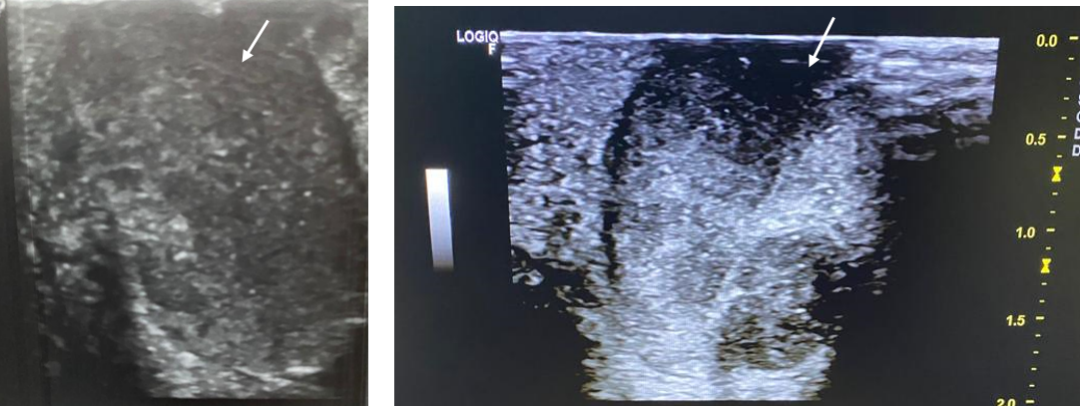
Ultrasound showing a penial hypoechoic and heterogeneous lesion.
